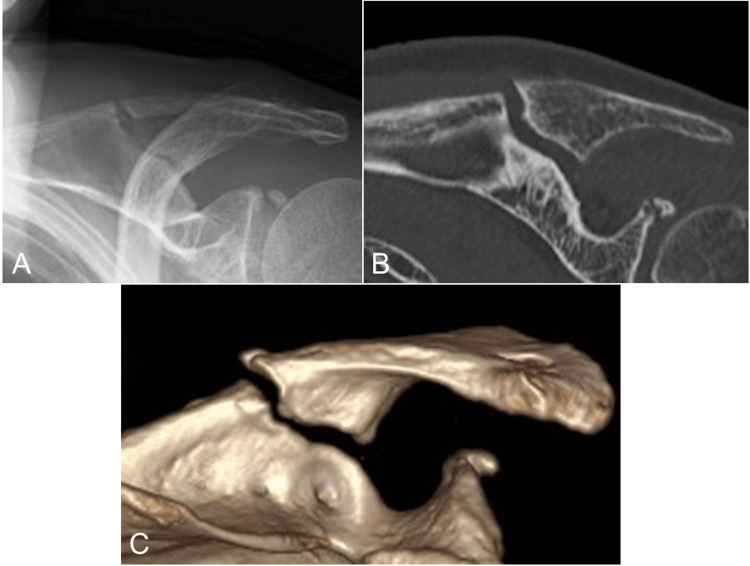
Radiological findings at 15 months after the injury Plain radiography (A) and CT (B, coronal view and C, anteroposterior view of 3D-CT) show the remaining dislocation of the fracture.
